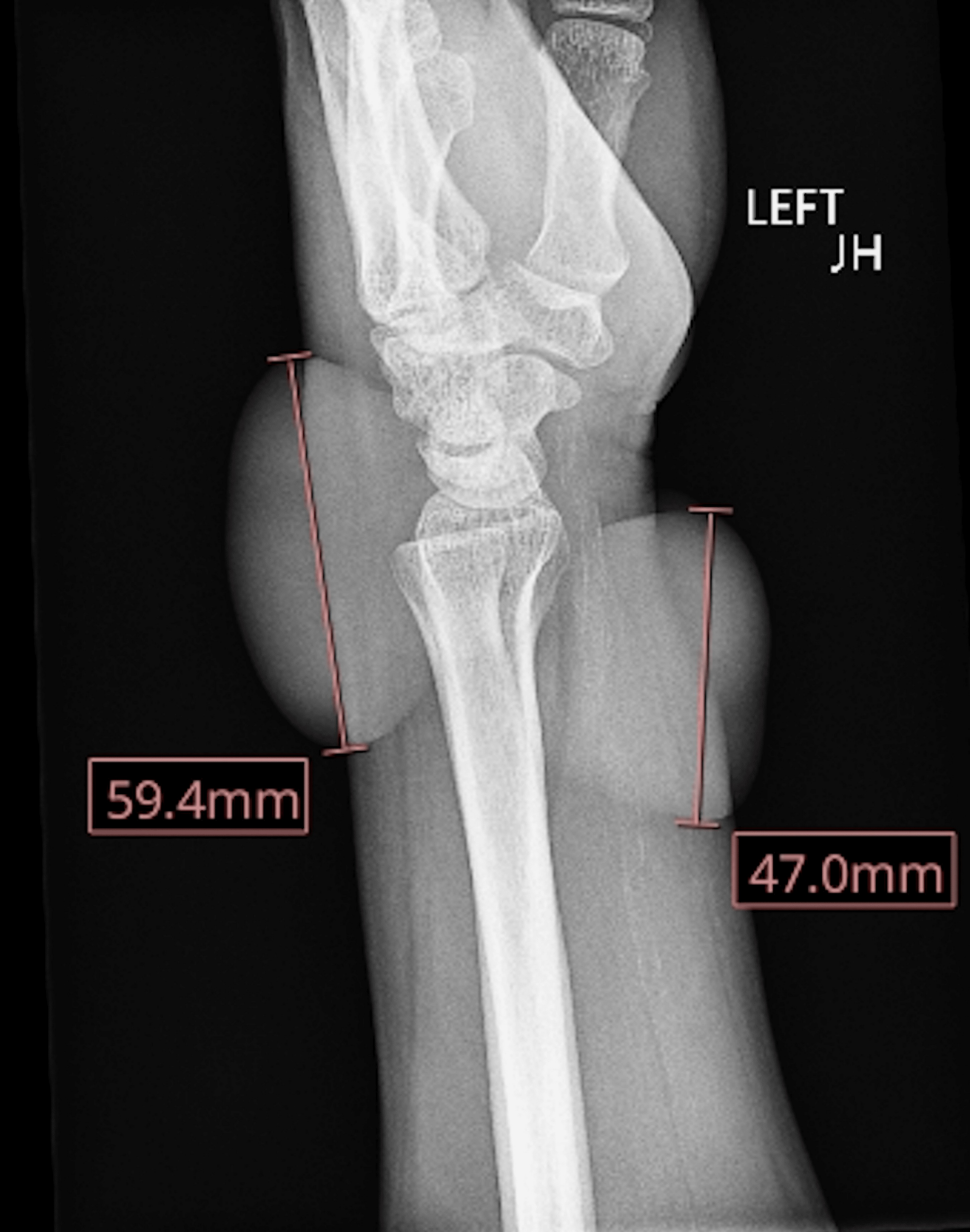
Lateral X-ray of the left wrist and wrist showing a lobulated lesion involving the dorsal aspect of the wrist and distal forearm consistent with the burn
